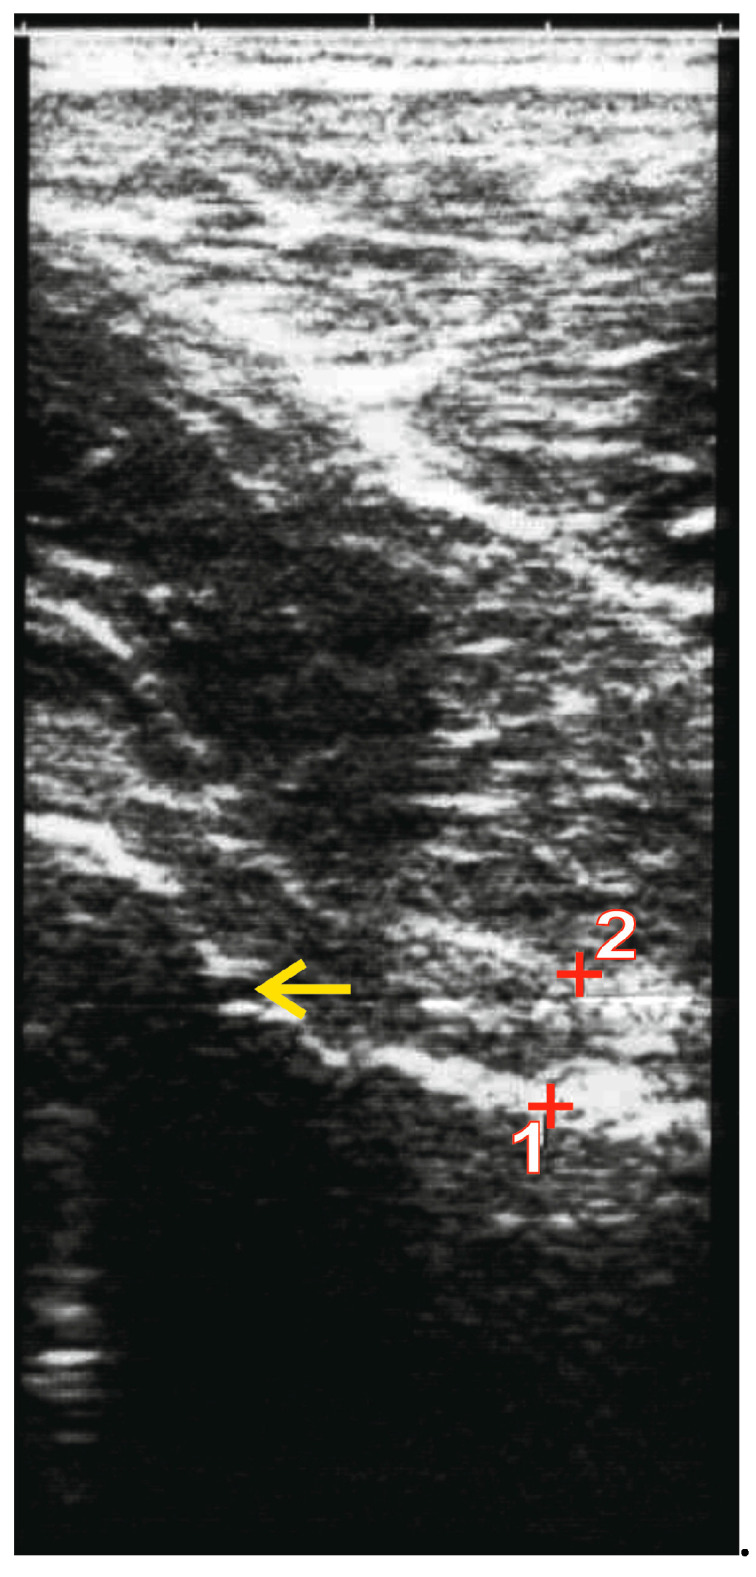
Ventral longitudinal section (parallel to femoral neck). Irregularity of cortical bone probably in the area of former epiphyseal joint (arrow) with disharmonious contouring of femoral head–neck connection. Distance between cortical bone of femoral neck (marker 1) and joint capsule (marker 2) of 5.8 mm is within normal range, with no intra-articular increase in volume. Ultrasound image was contrast-enhanced.
